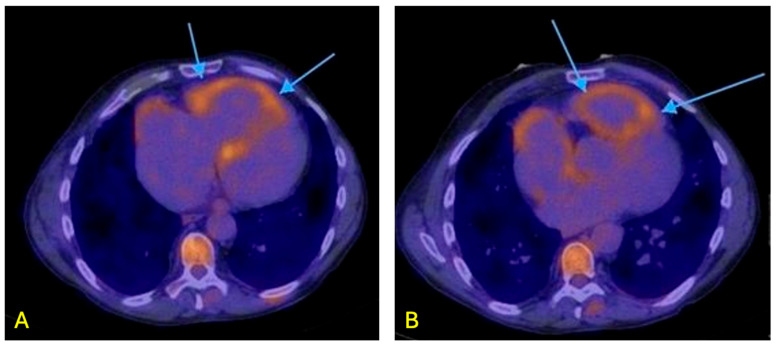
Cardiac PET with ^18^F-fluorodeoxyglucose (FDG) tracer. (A,B) Arrows showing extensive FDG uptake involving the entire right ventricle wall, the right atrium, and the interventricular septum with additional absence of left ventricle free wall FDG uptake. Reprinted/adapted with permission from Ref. [91].
